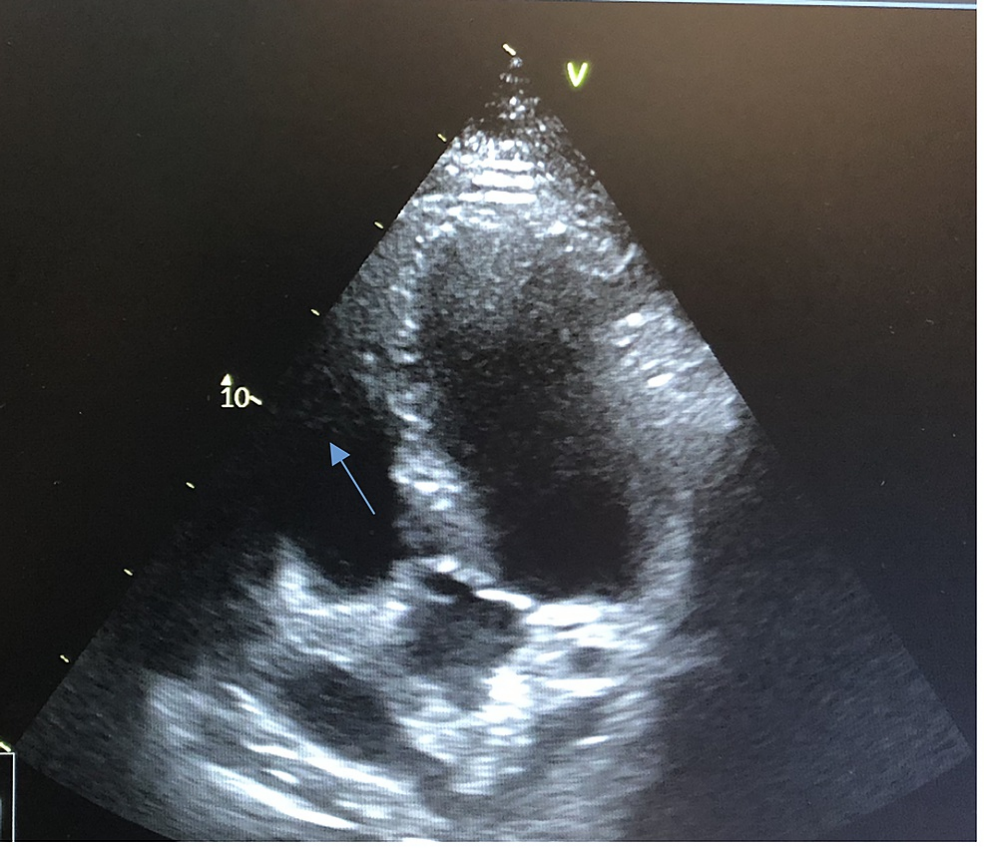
Echocardiogram showing no thrombus in the RV
